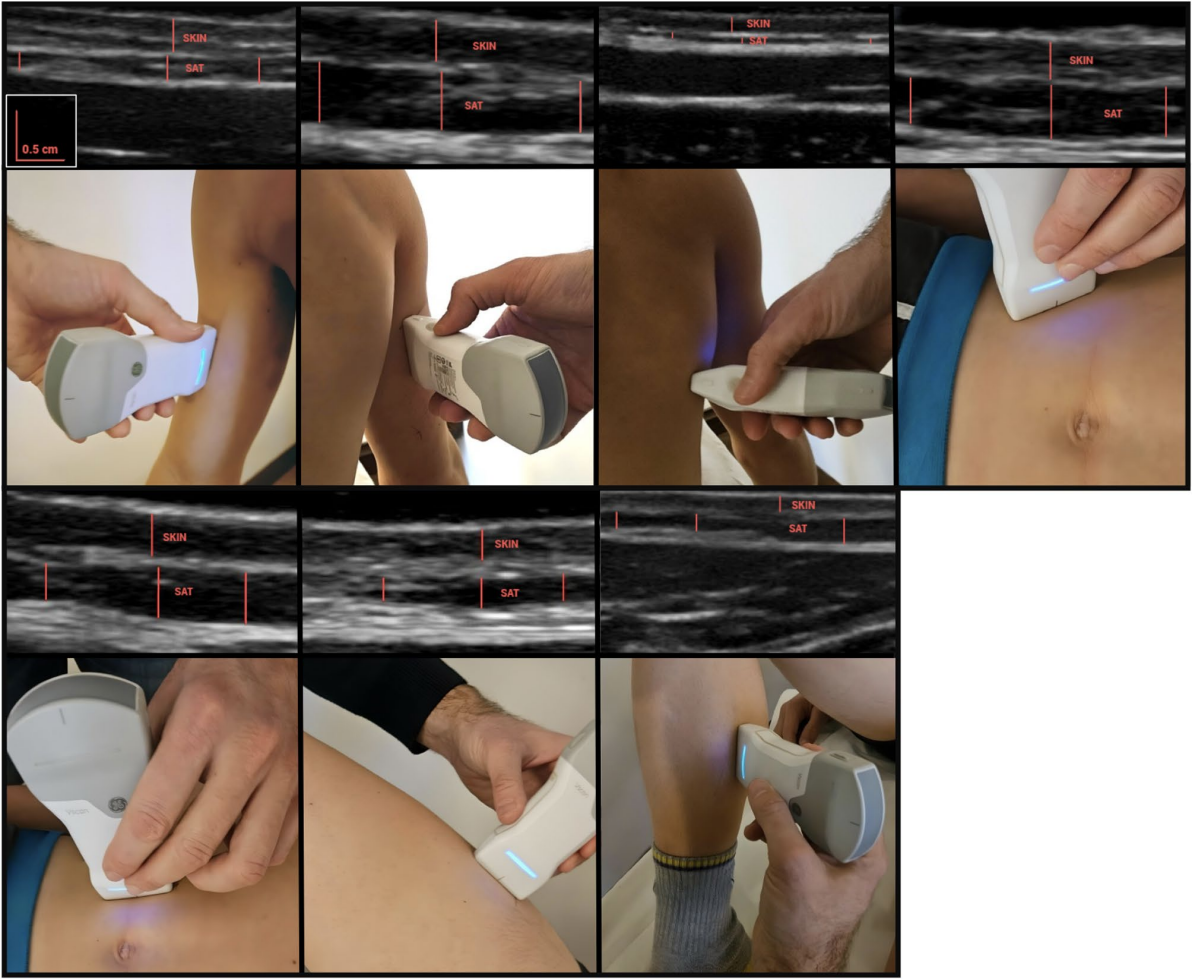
Sites of ultrasound measurements
